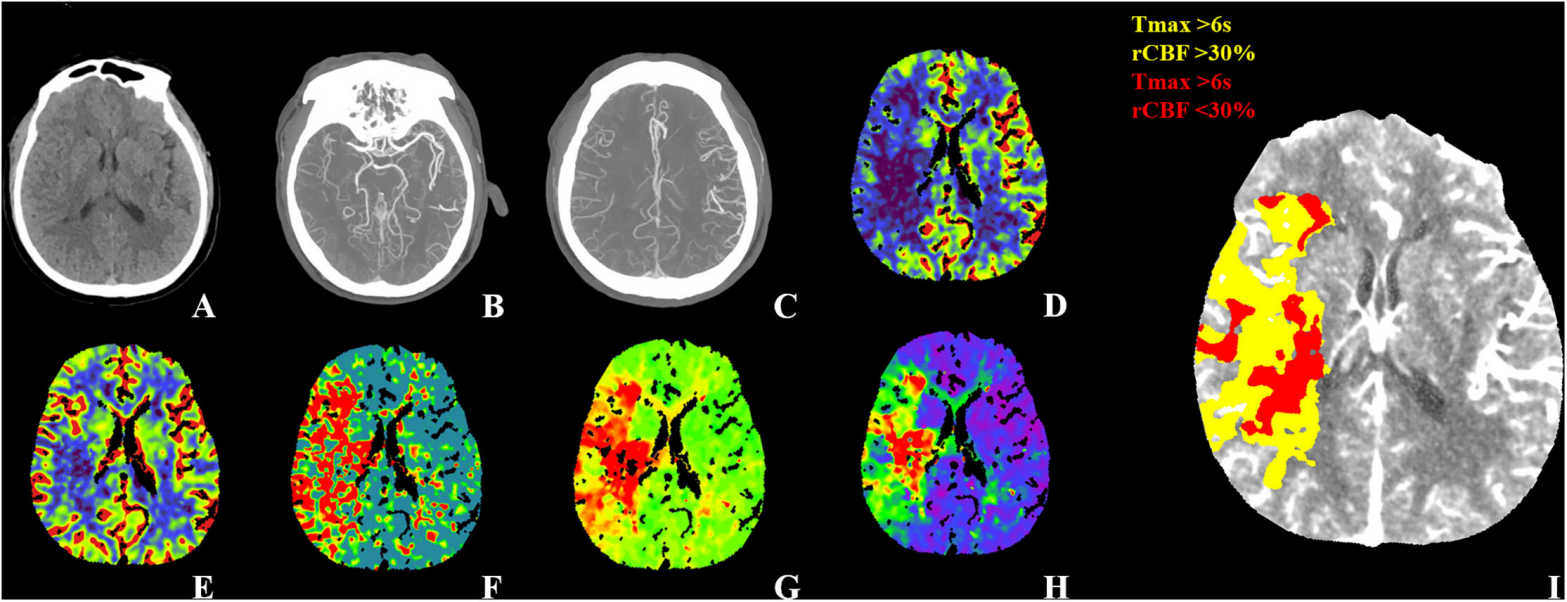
WUS with good outcome. Fifty-four-year-old male patient WUS patient last seen well 6.4 h before imaging. (A) The NECT didn't show any hypodensity with ASPECTS 10. (B) CTA-MIP showed right distal M1 segment occlusion with CBS 8. (C) CTA-MIP showed collateral filling nearly 100% of the MCA flow territory with collateral score three. The CTP showed (D) a large area with decreased CBF, (E) a smaller area with decreased CBV and (F–H) a large area with increased MTT, TTP, and Tmax. The CTP summary map (I) showed 76 ml of Tmax >6 s (yellow) and 34.93 ml of <30% rCBF decrease (red). The patient was treated EVT and showed good recovery with a 90-day mRS of one. WUS, wake-up stroke; ASPECTS, Alberta Stroke Program Early CT Score; MIP, maximum intensity projection; CBS, clot burden score; MCA, middle cerebral artery; EVT, endovascular thrombectomy; mTICI, modified Thrombolysis In Cerebral Infarction; mRS, modified Rankin Scale.
